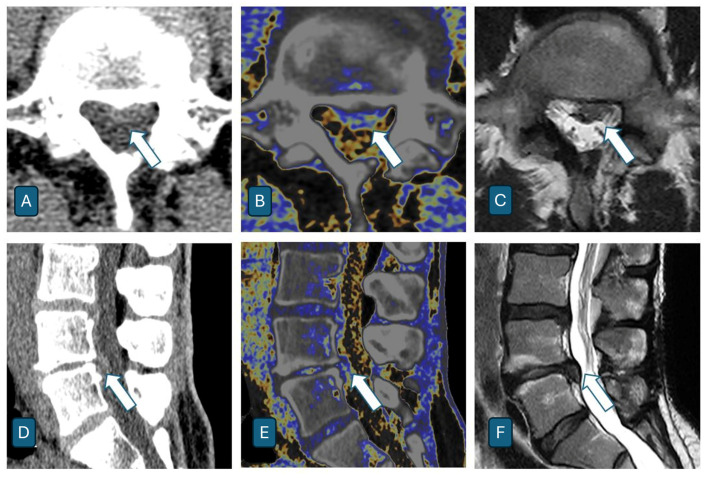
A 48-year-old female patient presenting with chronic midline lumbar pain. Axial and sagittal imaging reveal a predominantly central lumbar focal disk herniation with a small posterolateral left-sided component (white arrow). DECT images (B,E) provide a superior tissue contrast by employing a color-coded map that distinctly separates the herniated disk material (blue) from the surrounding bone and cerebrospinal fluid (displayed in grayscale). This colorimetric enhancement makes it easier to identify the herniation margins and differentiate them from adjacent structures, which is more challenging on conventional CT (A,D). Although MRI images (C,F) remain the gold standard for soft tissue evaluation, DECT represents a valuable imaging option, particularly when MRI is contraindicated or unavailable.
